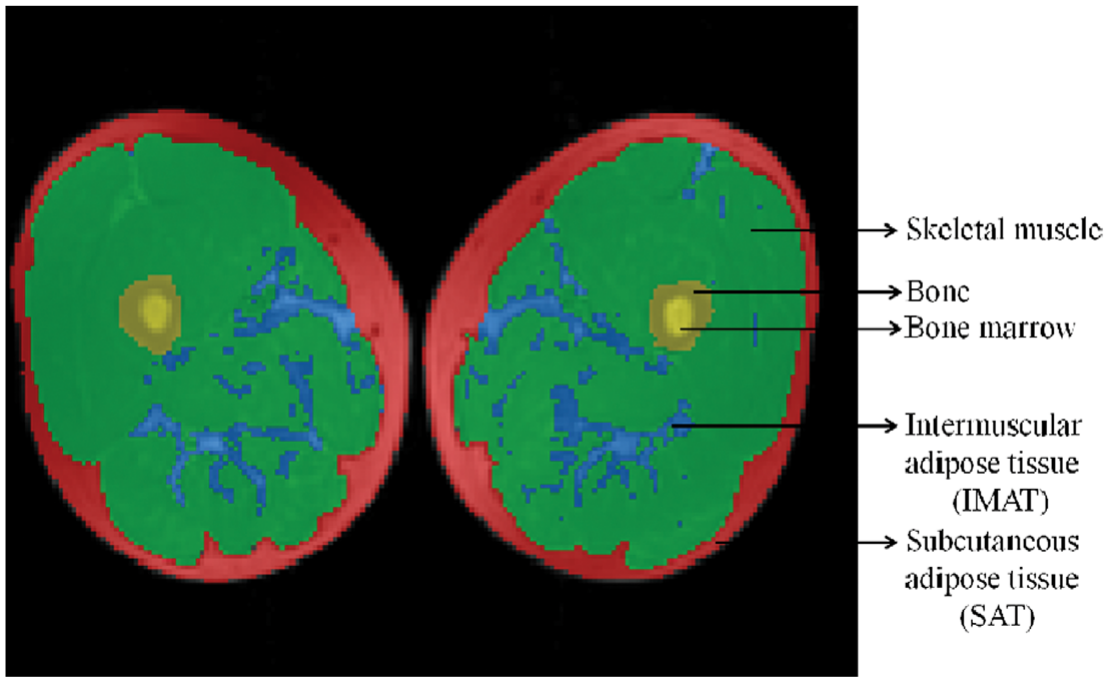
Segmentation of the original T1- weighted magnetic resonance image of legs. Subcutaneous adipose tissue (SAT), intermuscular adipose tissue (IMAT), skeletal muscle, bone and bone with marrow are shown.
